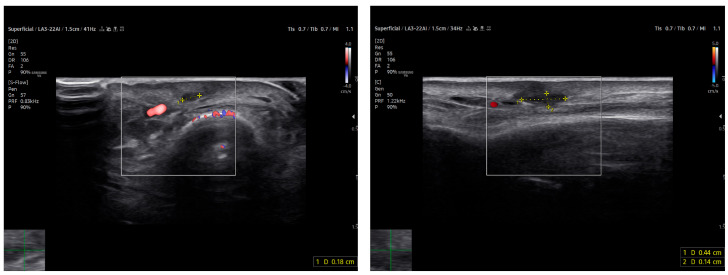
At follow-up, the ultrasound scan displays the area of the resected lesion and the scar region in both transverse (on the left) and longitudinal sections (on the right) above the long extensor tendon of the uninjured third finger.
